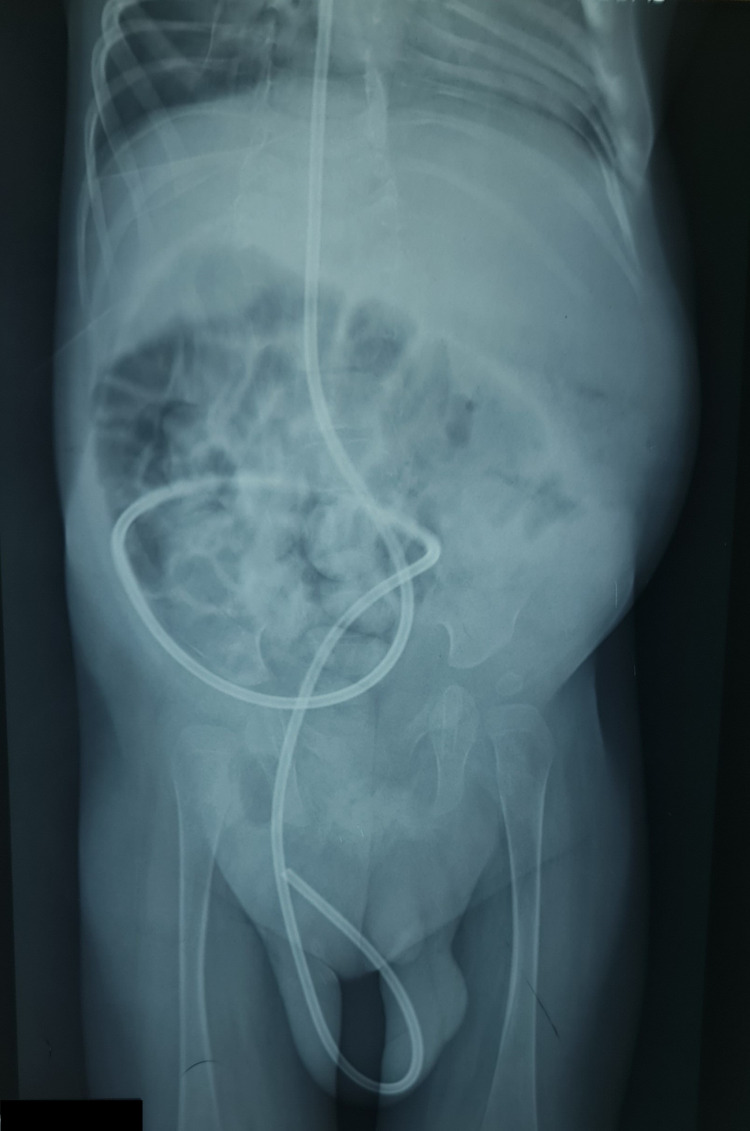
X-ray showing the lower end of the VP shunt passing into the right scrotum through the inguinal canal.
